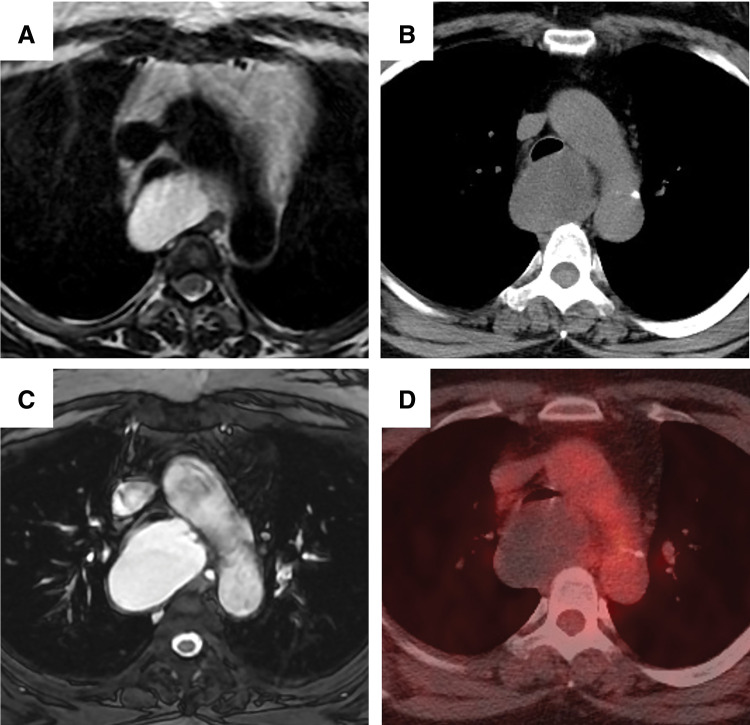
Radiological findings. (A) MRI performed 10 years prior to the surgery demonstrates a uniform cystic lesion. (B) Preoperative chest CT shows a well-defined mass in the middle mediastinum. (C) Preoperative T2-weighted MRI demonstrates a uniform high signal inside the mass. Compared with the MRI obtained 10 years earlier, the mass is enlarged, and the tracheal stenosis is more severe. (D) Preoperative PET reveals no accumulation within the mass.
